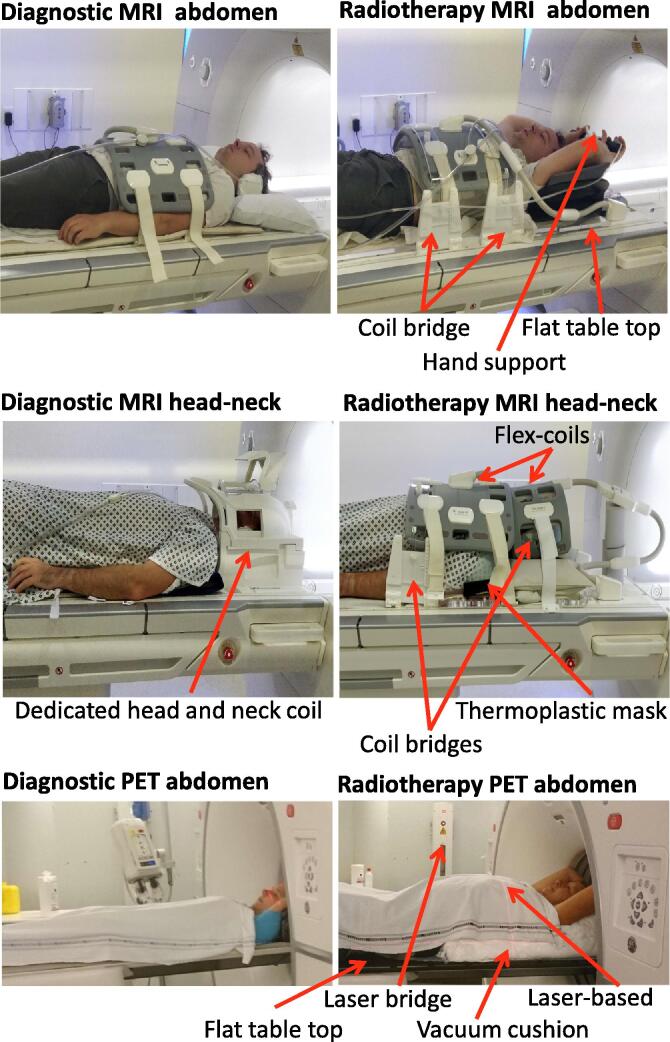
Diagnostic (left) and radiotherapy (right) setup for different modalities (MRI top two rows and PET bottom row) for different indications (from top to bottom: abdomen, head and neck, abdomen).
